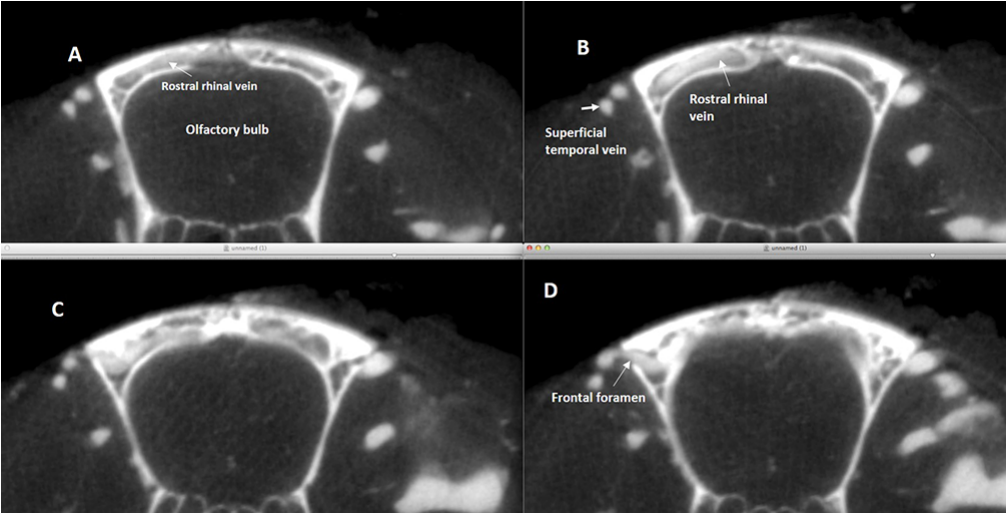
Axial silicon-microcomputed tomography of the rostral rhinal vein. (A-D) Four consecutive axial silicon- microcomputed tomography slices in cranio-caudal sequence showing the drainage of each rostral rhinal vein, which, after an intraosseus course, joins with the ipsilateral superficial temporal vein, through the frontal foramen.
